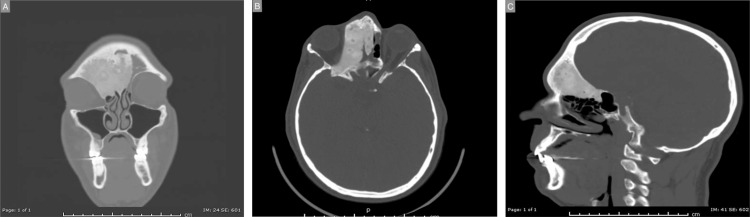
Preoperative CT. A: Coronal. B: Axial. C: Sagittal.
